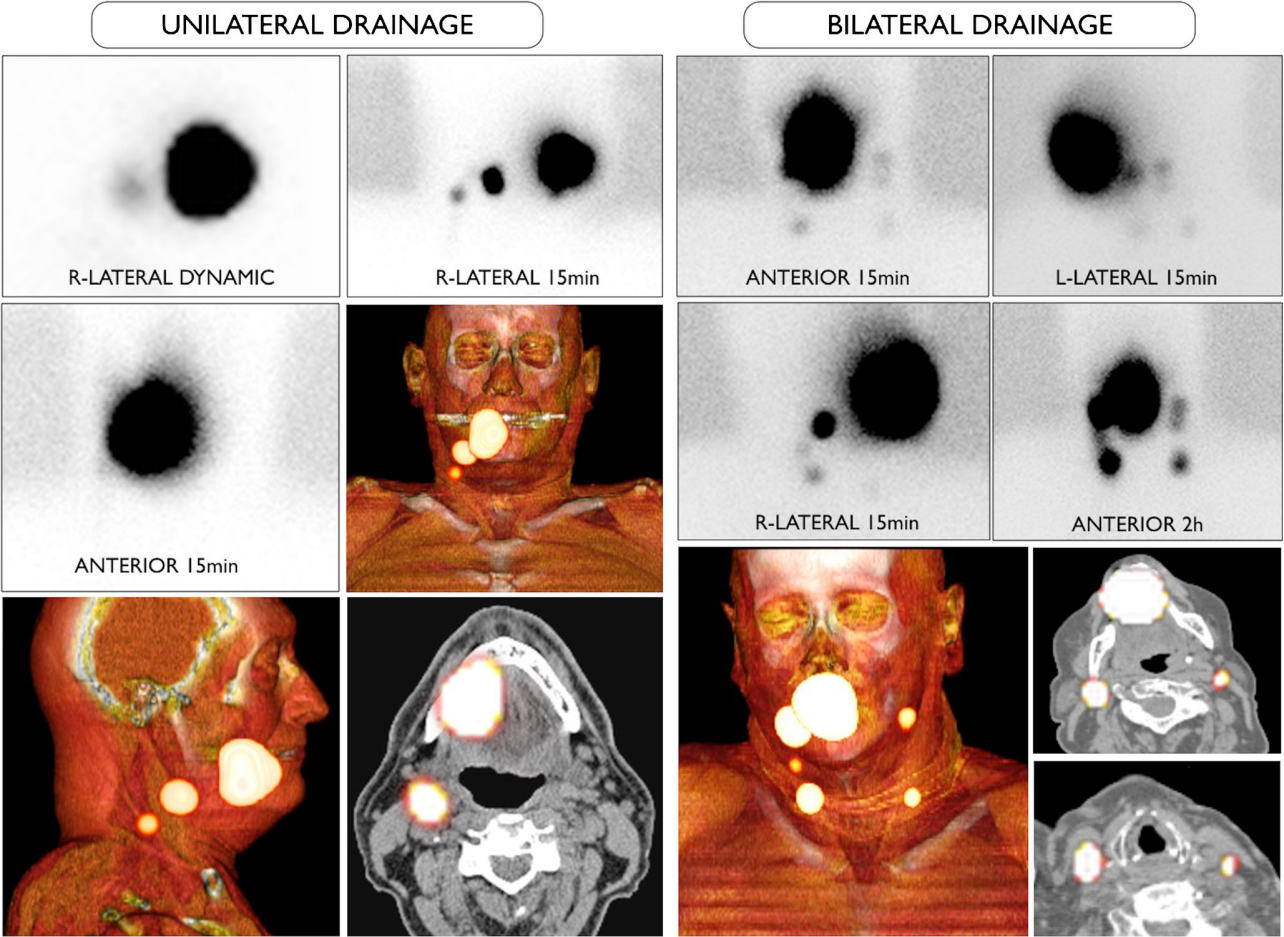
Lymphatic drainage to the neck is unilateral in a 55-year-old male patient with a T1 primary tumour localized on the right side of the tongue (on the *left*) and bilateral in a 72-year old female patient with a T1 midline tongue carcinoma (on the *right*). Note that in both cases the sternocleidomastoid muscle, as depicted on volume rendering and cross-sectional SPECT/CT, is an excellent landmark to anatomically refer the location of sentinel lymph nodes in relation to lymphatic basin and surgical neck level
